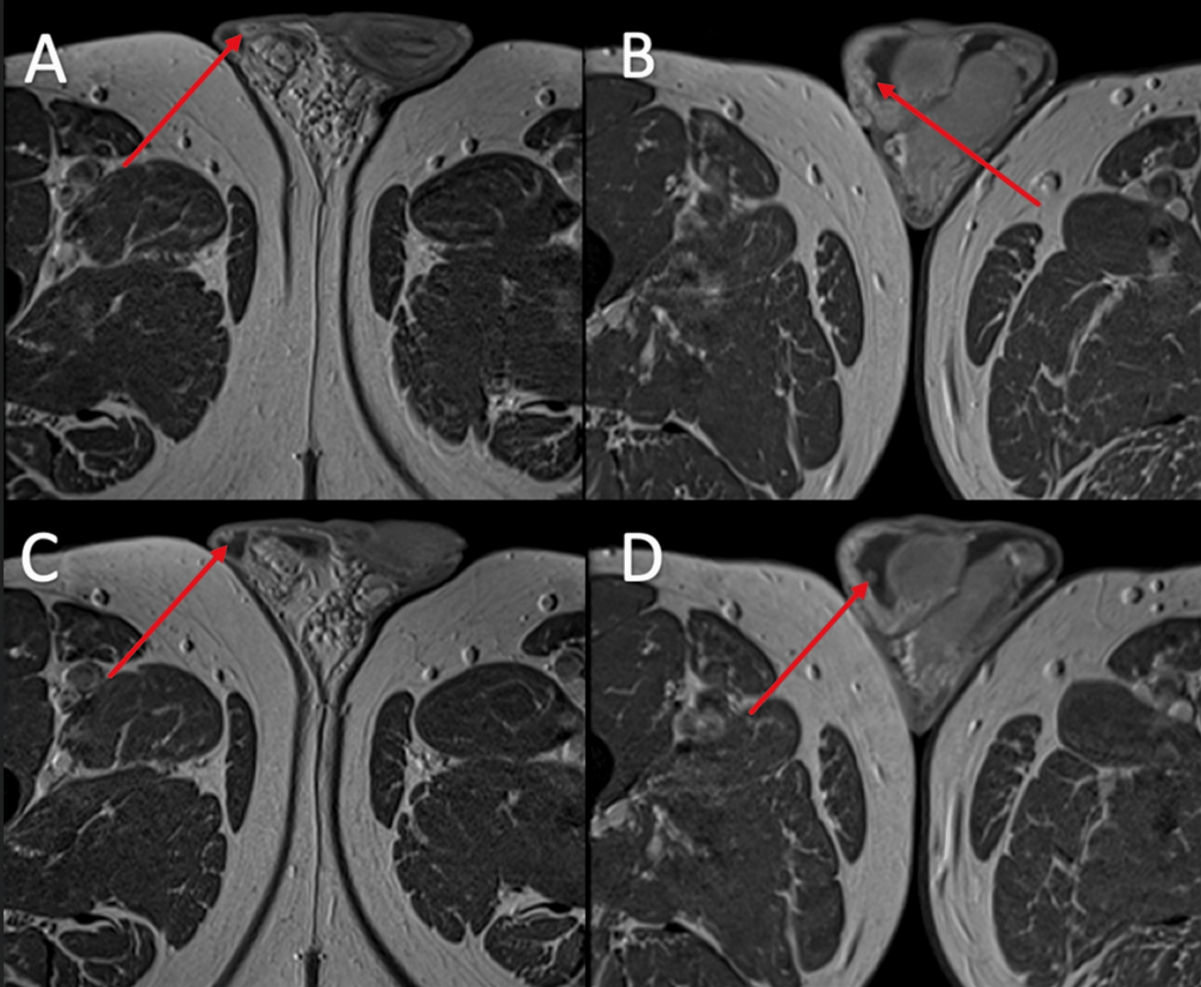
Post-gadolinium contrast T1-weighted axial MRI Peripheral contrast enhancement of nodules (A,C), with general enhancement of smaller nodules (B,D).
